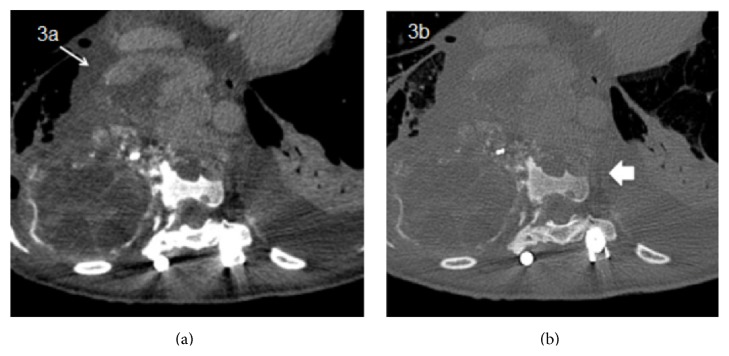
Postcontrast CT on soft reconstruction filter (a) and hard reconstruction filter (b), showing a lytic bone lesion arising from the vertebral body (wide arrow) with a large soft tissue component (narrow arrow).
